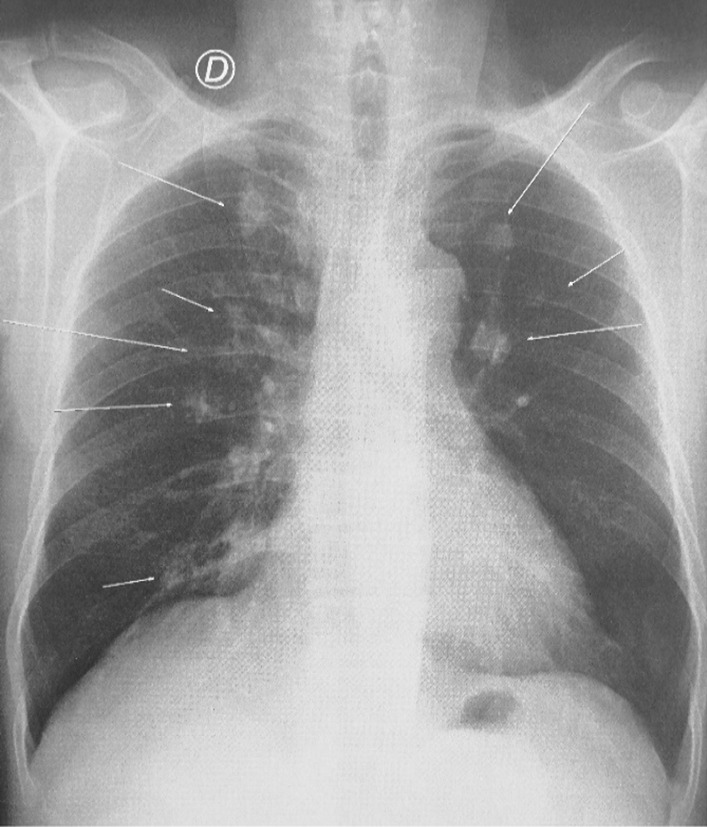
The frontal chest X-ray shows several excavated opacities
